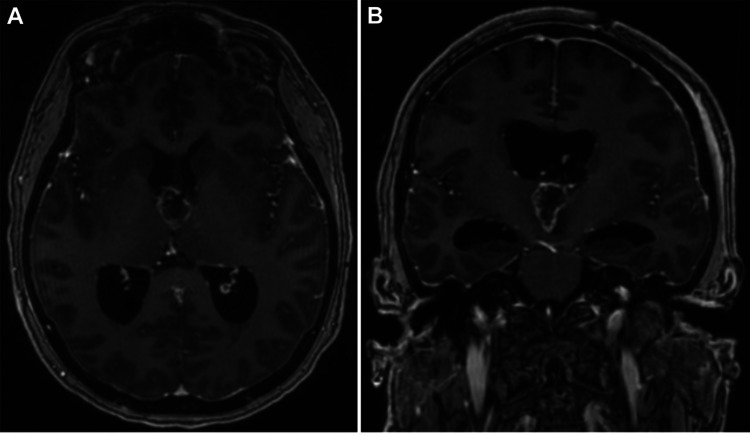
Postoperative Imaging Postoperative T1-weighted MRI showing peripheral enhancement throughout the resection cavity (A: axial and B: coronal).
